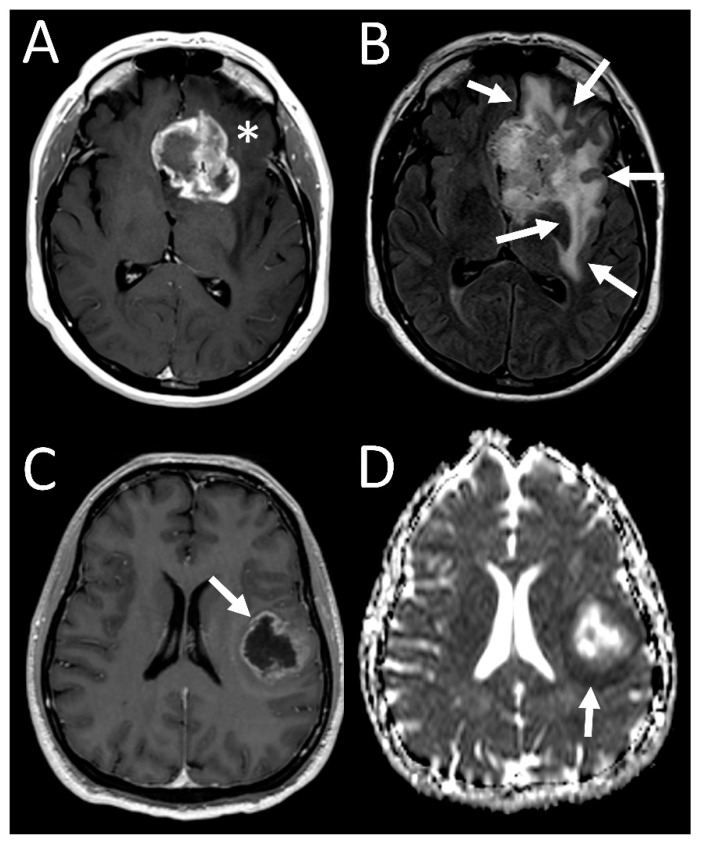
Sample imaging features of glioblastoma with 19/20 co-gain. (A) T1 postcontrast image of left frontal tumor demonstrating avid enhancement (asterisk), with (B) FLAIR image showing a large area of surrounding edema (arrows). (C) T1 postcontrast image of another tumor shows thinner rim of enhancing tumor (arrow), with (D) ADC map showing an area of non-enhancing, diffusion-restricting tumor beyond the enhancing tumor (arrow).
